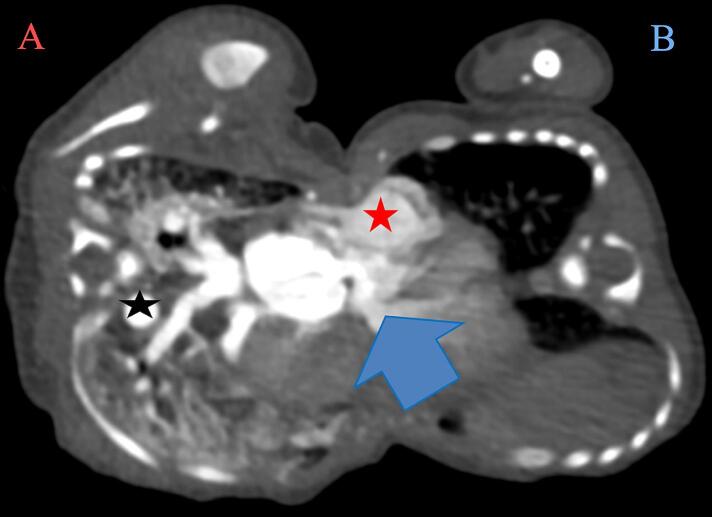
A contrasted CT scan in axial views with twin A on the left and twin B on the right. Twin A has a left-sided right ventricle (red star) with communication to the heart of twin B (blue arrow) and a right-sided descending aorta (black star). There is marked engorgement of the pulmonary vessels of twin A (increased lung parenchymal enhancement).
